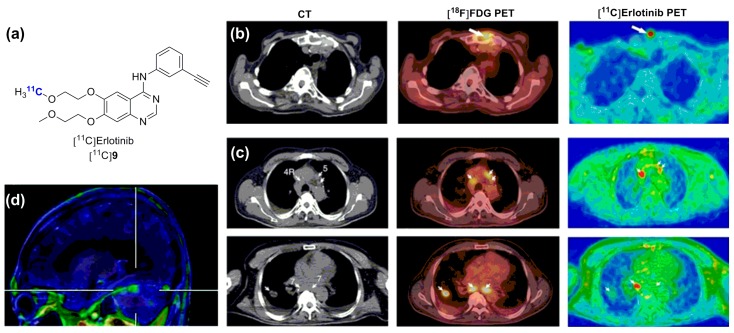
Selected *in vivo* results with [^11^C]erlotinib. (a) Chemical structure of [^11^C]erlotinib and comparative PET imaging studies; (b) Bone metastasis (c) and tumor/lymph node metastasis accumulation of [^11^C]erlotinib in non-small-cell lung carcinoma (with CT and [^18^F]FDG PET) (Figures in (b,c) adapted by permission from Macmillan Publishers Ltd on behalf of Cancer Research^©^, UK, [70] 2011); (d) Magnetic resonance imaging-PET image from [^11^C]erlotinib accumulation in brain metastatic lesions in a patient diagnosed with a non-small-cell lung carcinoma. (CT: computed tomography) (Adapted by permission from [71], from the publisher Wolters Kluwer).
